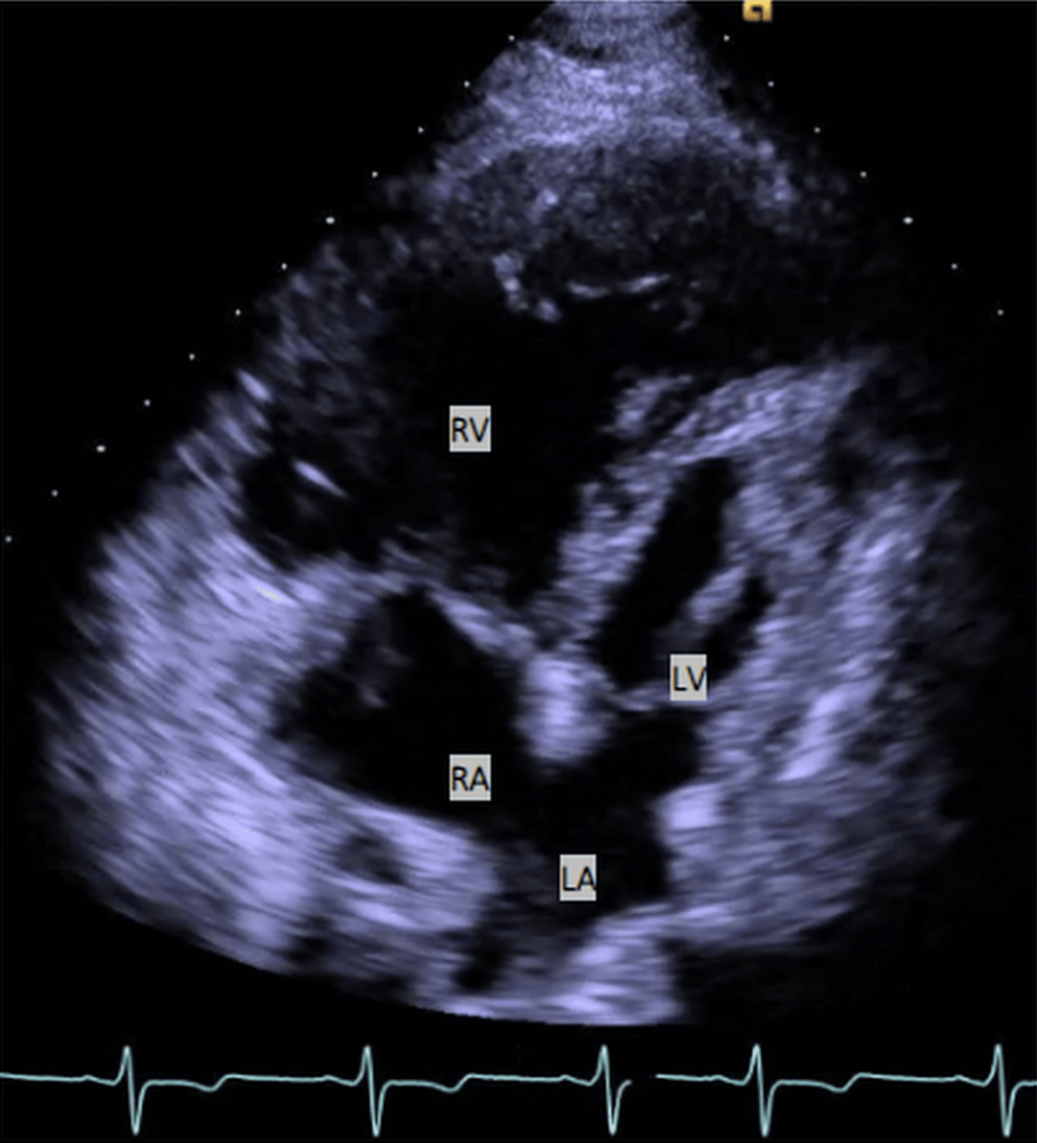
TTE showcasing an apical four-chamber view in a patient diagnosed with HLHS. The image highlights the classically hypertrophied systemic RV and the significantly underdeveloped LV. Additionally, the atria are visible, with a nearly absent septum dividing them - a result of a prior atrial septectomy. TTE, Transthoracic echocardiogram; HLHS, Hypoplastic left heart syndrome; RV, Right ventricle; LV, Left ventricle; RA, Right atrium; LA, Left atrium
